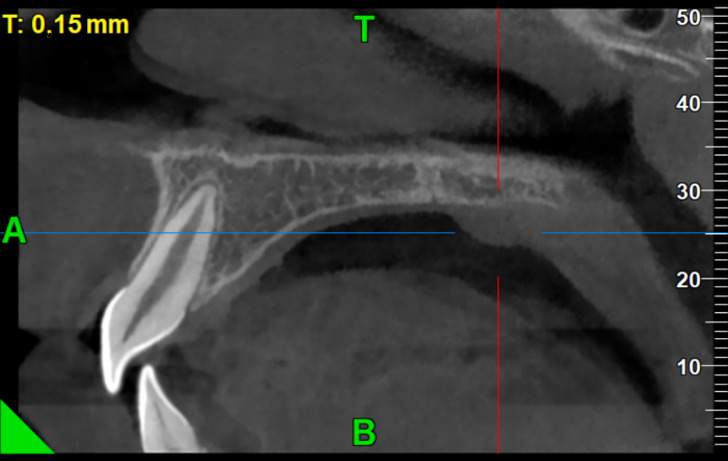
CBCT examination of the area under investigation. The lesion appears to be restricted solely in soft tissues.
